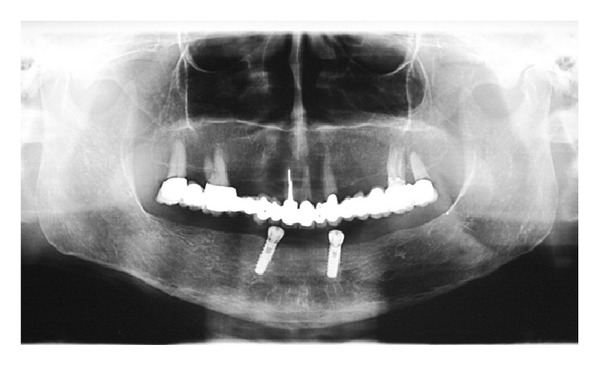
Panoramic radiograph showing the implants placed in the parasymphyseal region of the mandible. Implant supported prosthesis are particularly indicated in postoncologic patients with an altered oral anatomy.
